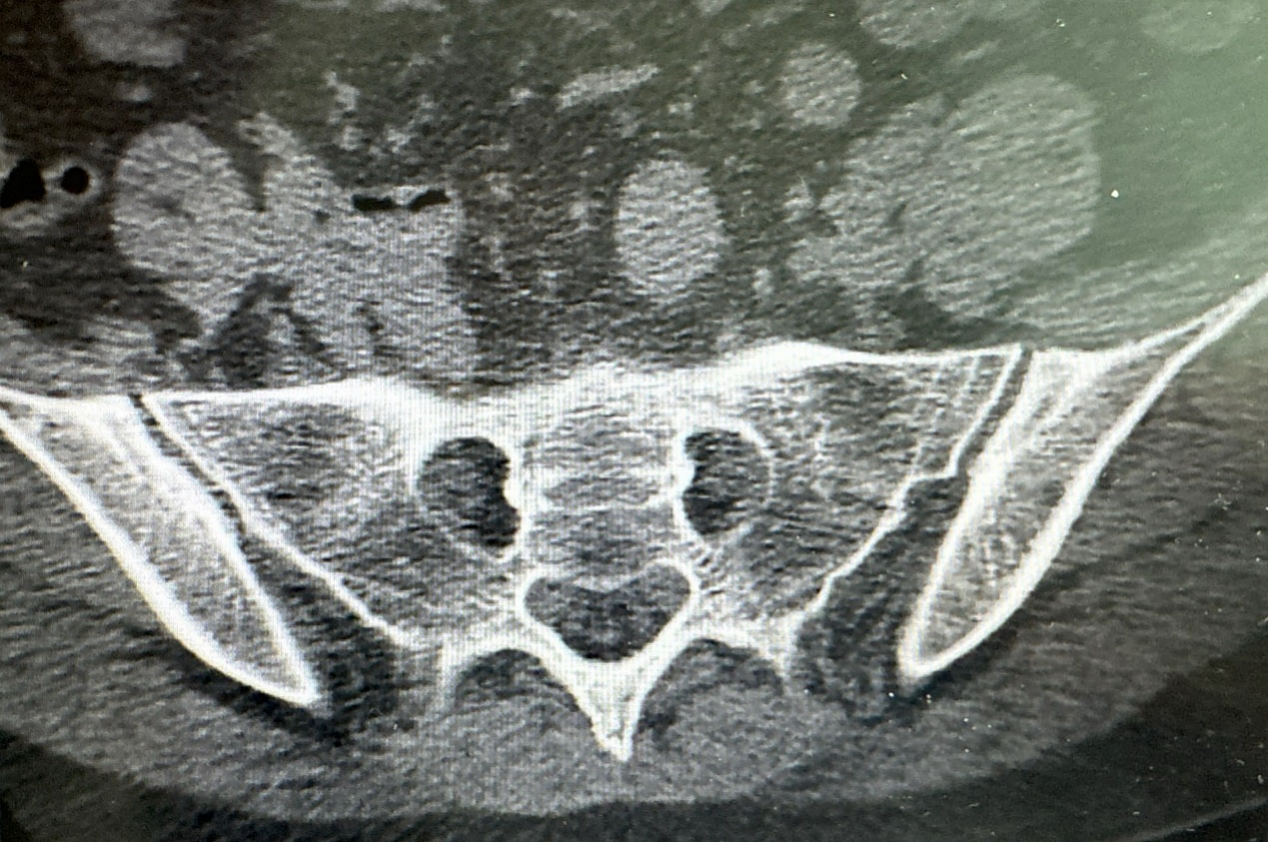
Joint CT shows findings suggest likely bilateral osteitis condensans ilii.
